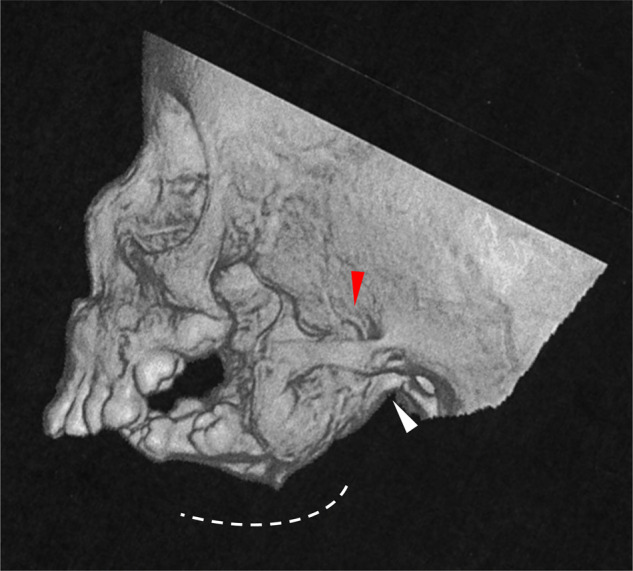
Three-dimensional computed tomography (3D-CT) image of the patient. Mandibular condyle agenesis and excessively hypoplastic rami and processes can be observed. The possible positions of the mandibular jaw (white arrowhead) and process (red arrowhead) are indicated. The mandibular angle is unclear
